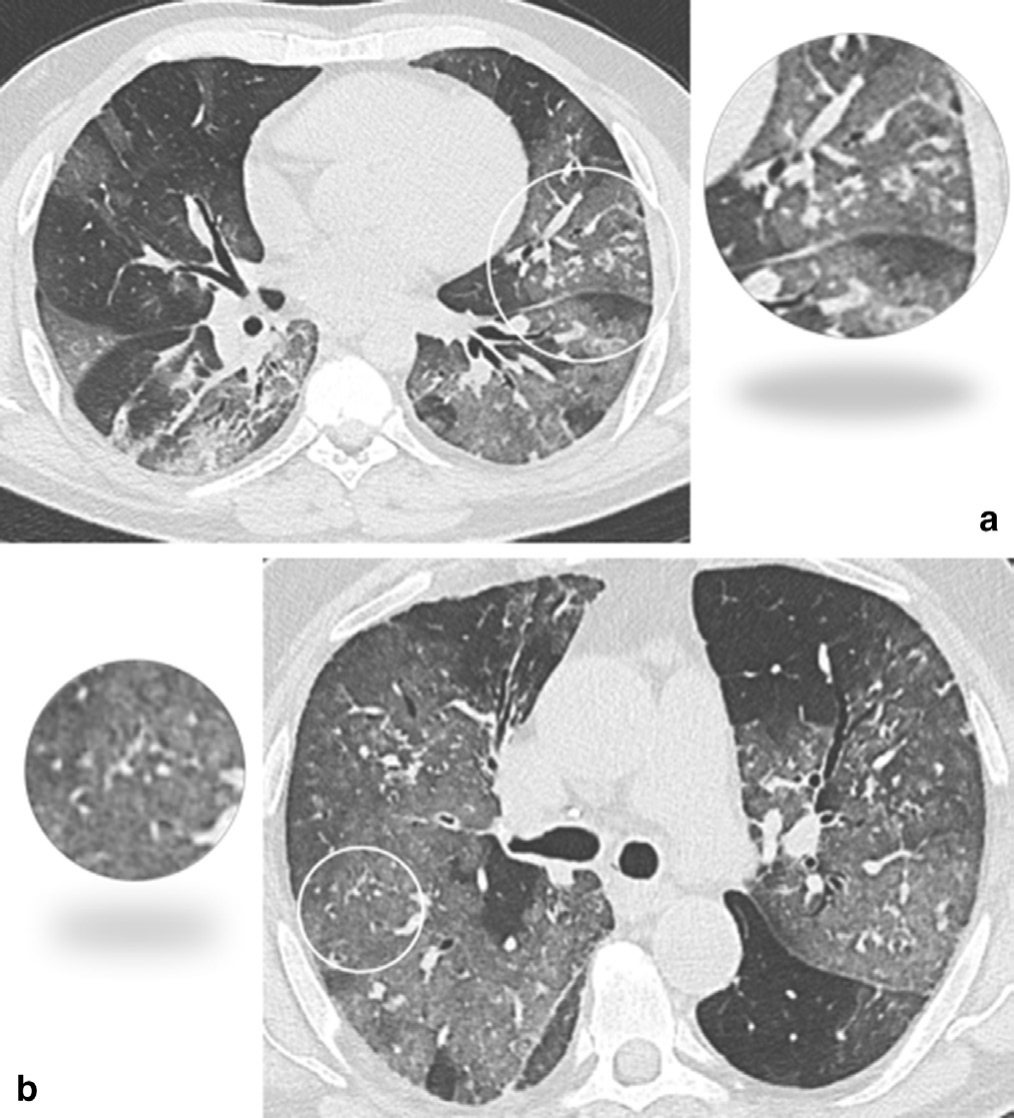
(a, b) Axial CT image without contrast showing solid centrilobular nodules (open circle and magnified vignette).
